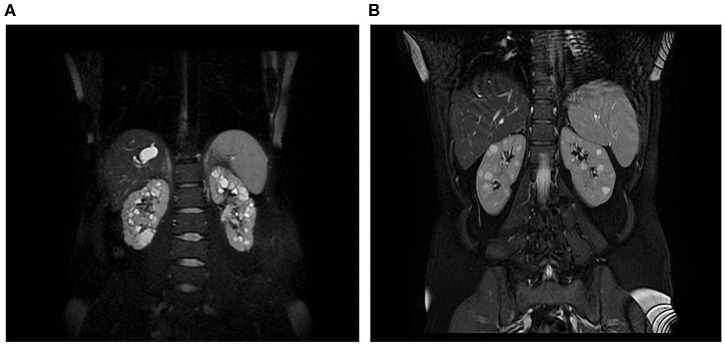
Kidney and liver images of two patients. (A) MRI of a 31-year-old woman with compound heterozygous mutations in *PKHD1*, who received a diagnosis of polycystic kidneys at 17. (B) MRI of a 45-year-old woman, who carried two heterozygous variants in *PKHD1*, p.(His3124Thr), and *PMM2*, p.(Gly42Arg).
